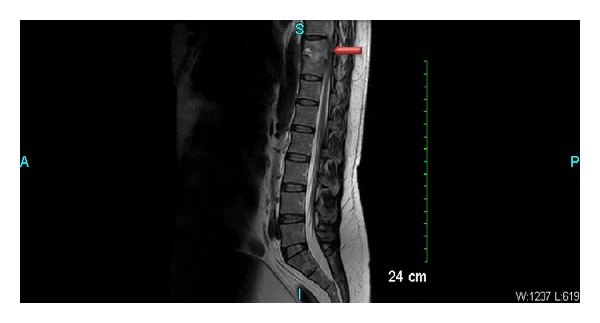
MRI of lumbosacral spine showing discitis at T10-T11 level (red arrow).
